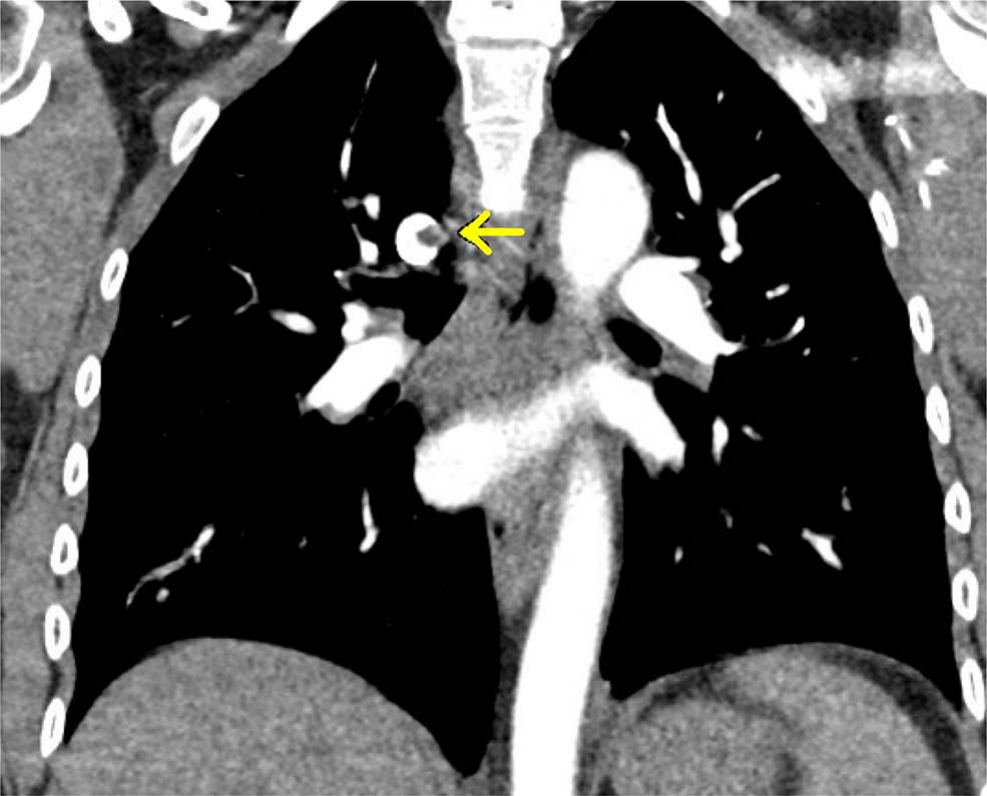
CTA chest (august 2024) demonstrating azygos vein medial wall thrombosis.
